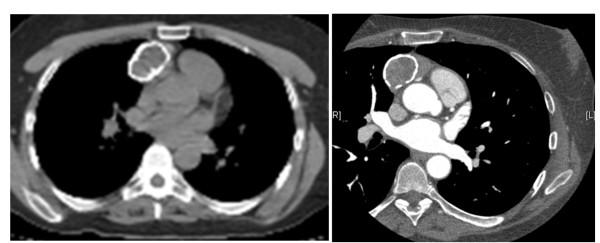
Computed tomography (CT) scan showing a 3.9 cm rim calcified right anterior mediastinal mass. CT with intravenous contrast (right panel) shows the mass in relation to mediastinal vasculature.
